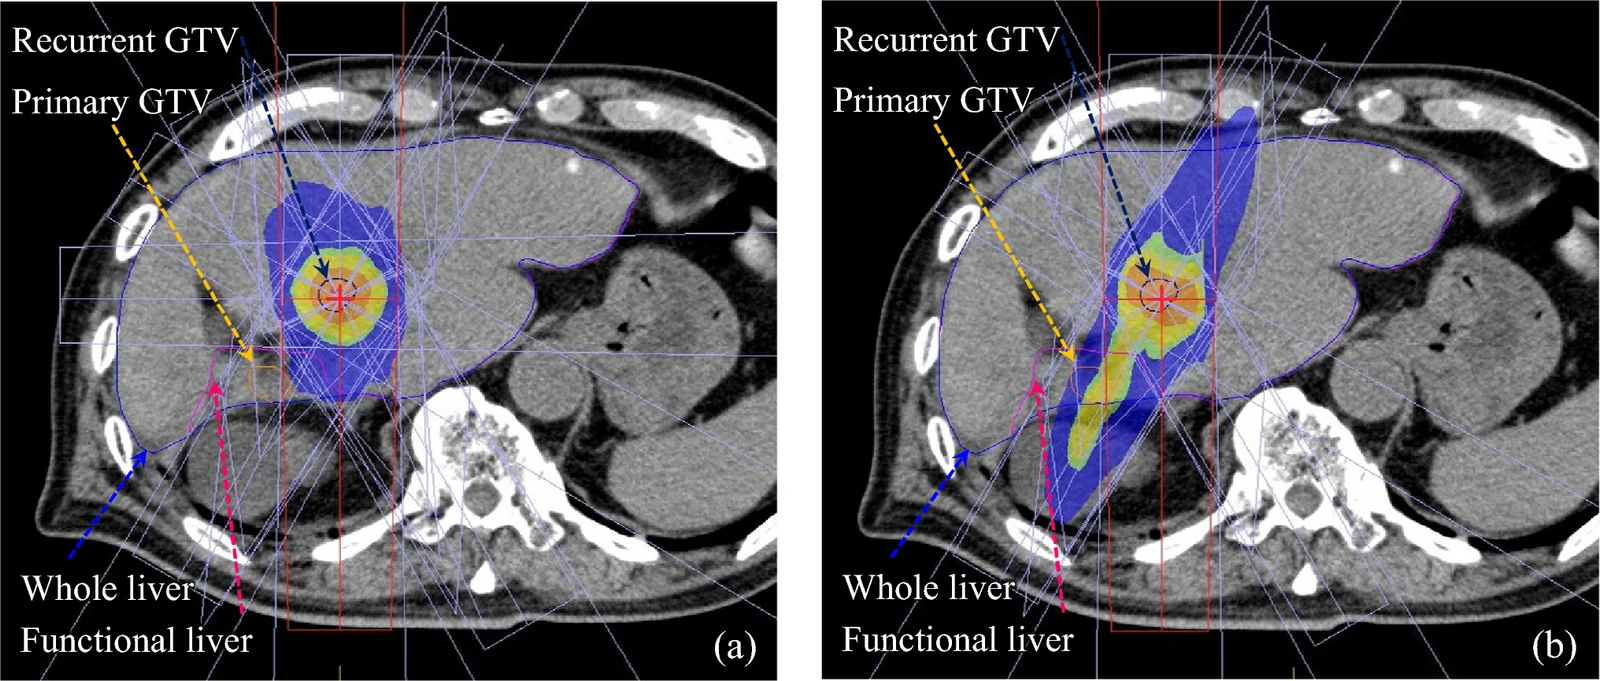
Comparison of beam arrangements and dose distributions between conventional and functional image–guided planning. a Conventional plan without functional liver information. b Functional image–guided plan incorporating ^99m^Tc-GSA SPECT. The primary lesion (previously irradiated region), recurrent target lesion, whole liver, and functional liver are shown. Although the primary lesion is located within the anatomical liver contour, it is excluded from the functional liver contour due to reduced ^99m^Tc-GSA uptake following prior SBRT. In the functional image–guided plan, beam arrangement weighting was applied to reduce irradiation to regions with preserved hepatic function
